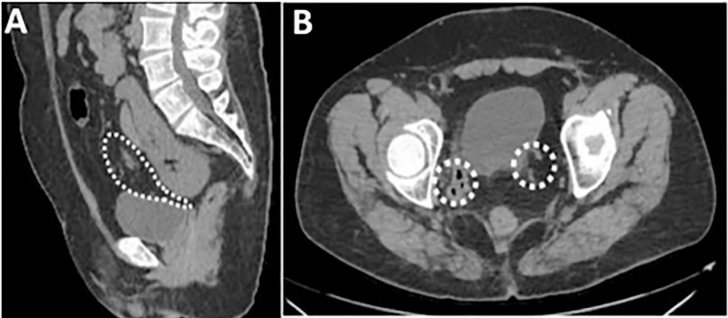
Internal sexual organs: (A) CT sagittal section, showing the absence of uterine (B) CT axial section, 28*27mm axial section, which reinforces that it is located in the contour of the cecum, compatible with ovarian tissue.
